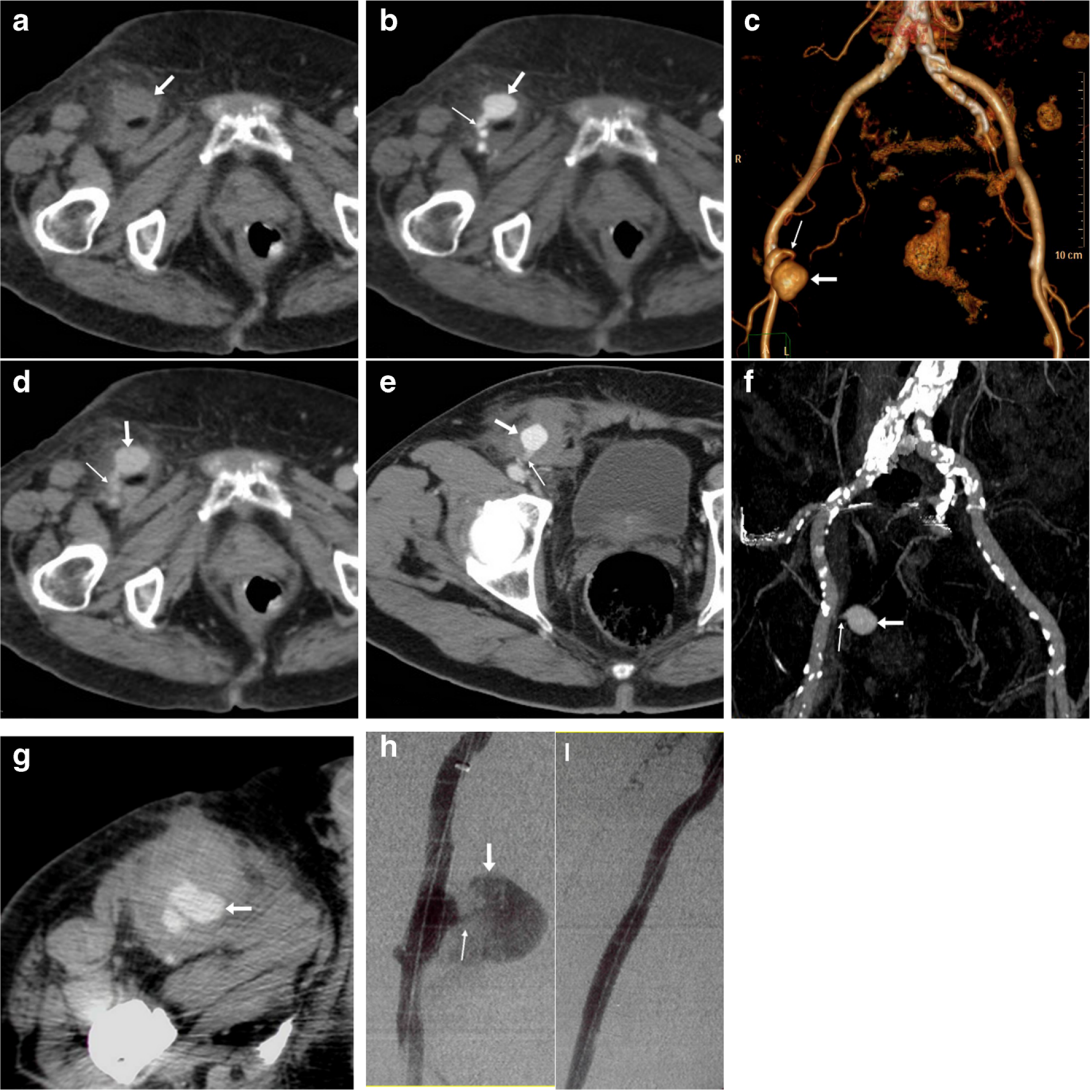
Three cases of right CFA pseudoaneurysms (PA). A-D) In an 87-year-old diabetic woman suffering from blood loss following PCI precontrast (A), CT angiography (B, volume-rendering C) and venous-phase (D) images showed small-sized (2 cm) round-shaped PA (arrows) and a short feeding tract (thin arrows) communicating with the CFA. E-F) In a 75-year-old man treated with thoracic endovascular aortic repair, CT-angiography (E) and MIP reconstruction (F) showed characteristic appearance of a PA (arrows) surrounded by blood, without active CM extravasation. Both occurrences were treated conservatively. G-I) In a 70-year-old man, CT angiography (G) showed a 5-cm right CFA PA (arrow), which was then confirmed angiographically (I) including optimal visualisation of thin connecting tract (thin arrows) and successfully treated with covered stent placement (I)
